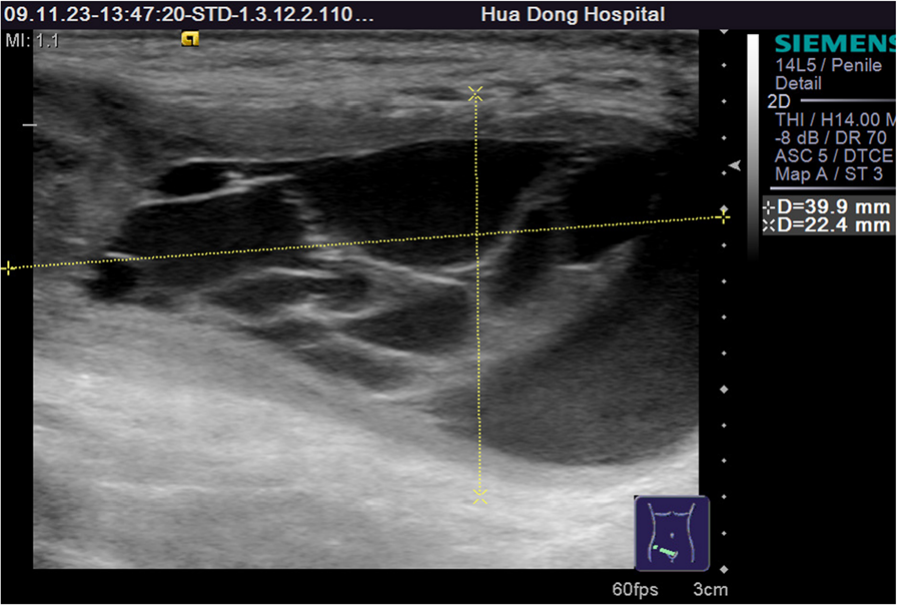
The seroma showed in the ultrasonography. The multilocular seroma was visible in front of the mesh
